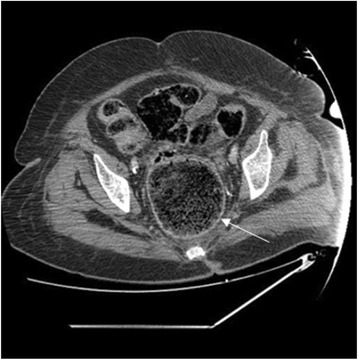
Pelvic CT scan showing a huge fecal impaction with thickened and edematous rectal wall (*arrow*)
